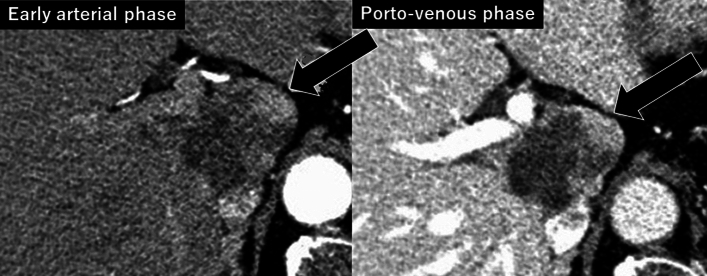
After LEN-TACE treatments for 7 months, HCC in the segment 1 was shrink as 5.5 cm and tumor compression was released but only posterior portal pedicle was involved as a stenosis by the follow-up CT. The tumor vascularity was almost disappeared at the arterial phase (black arrow)
